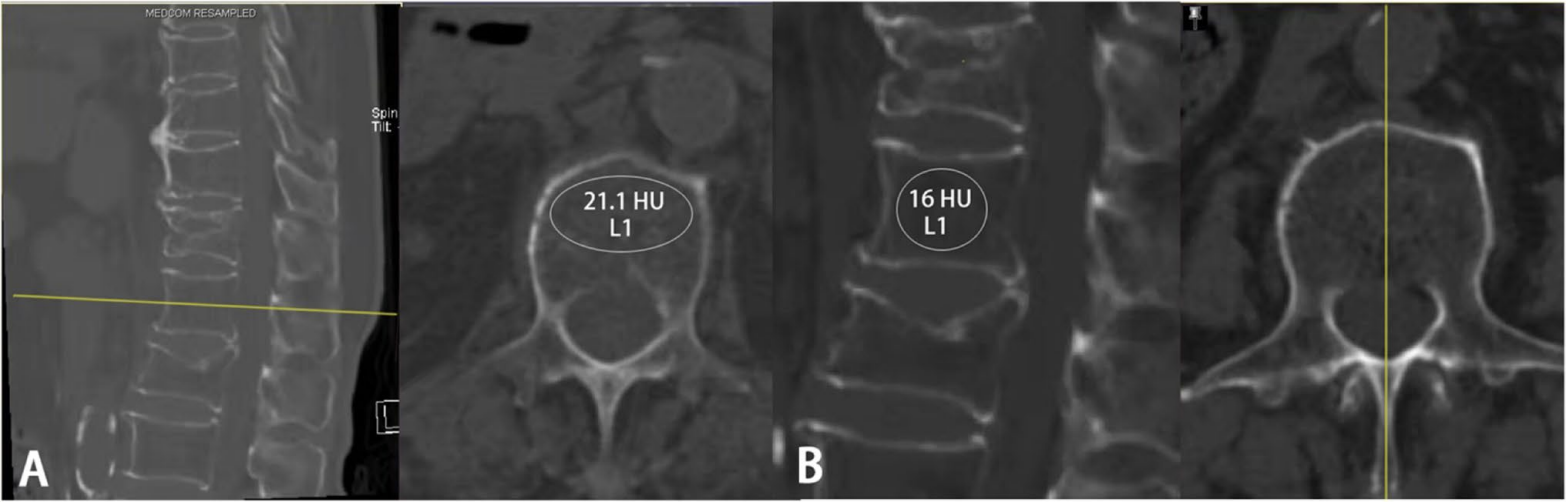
Example of CT HU measurement: when drew an oval region of interest (ROI) over the axial (A) and sagittal (B) of L1 mid-body, PACS software automatically calculates the average CT HU for the region of interest. These were the CT images of a 77 years old female patient who suffered a T12 OVCF; the axial and sagittal L1-HU values were only 21.1 HU and 16 HU, respectively. She suffered a second fracture of L1 after only 2 months
